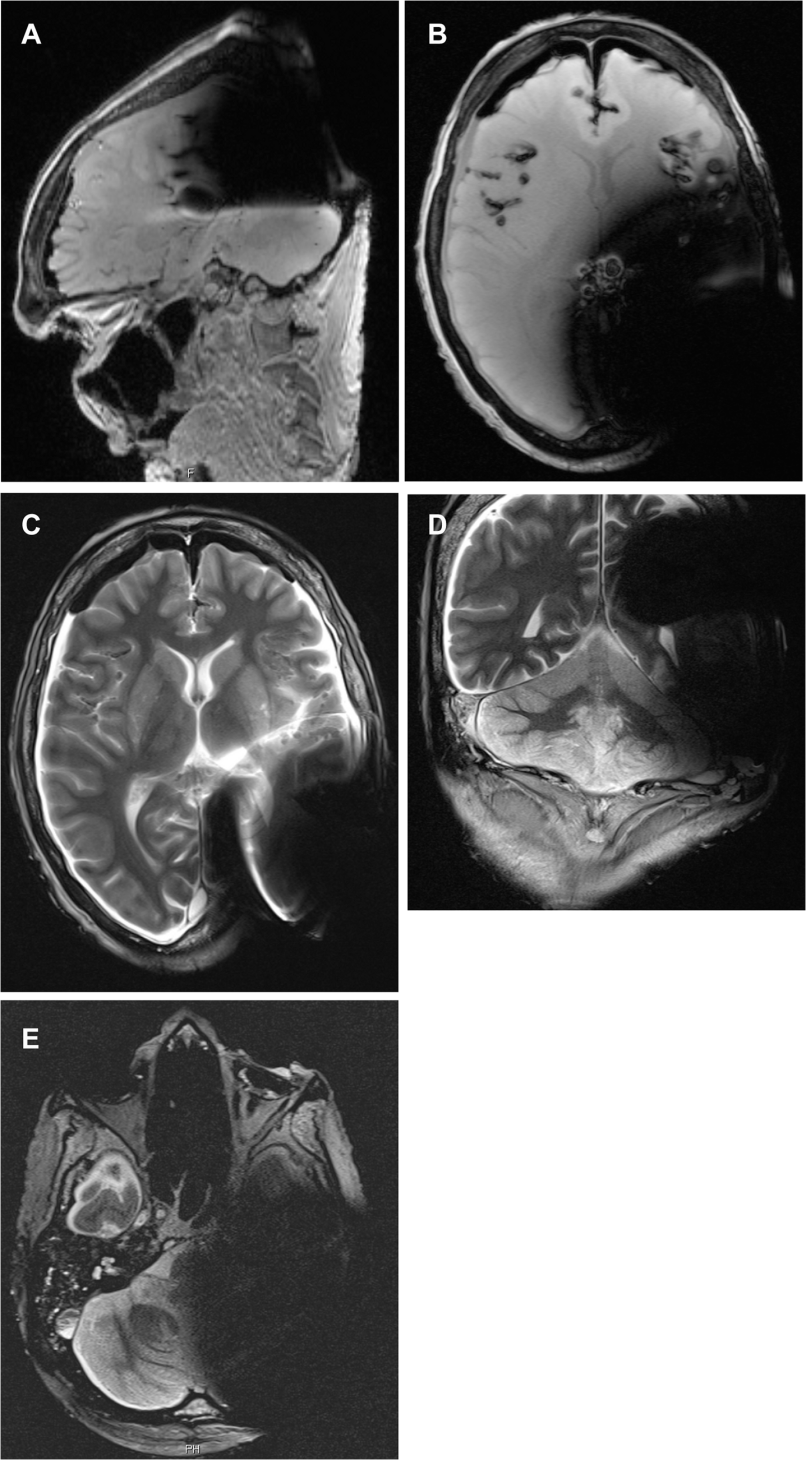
MR image series of one corpse head at 3T with magnet in situ. 3a: T1w MPR sagittal; 3b: T1w axial; 3c: T2w axial; 3d: T2w coronal; 3e: CISS. The MR images show the maximum in diameter of the artefact in each sequence.
